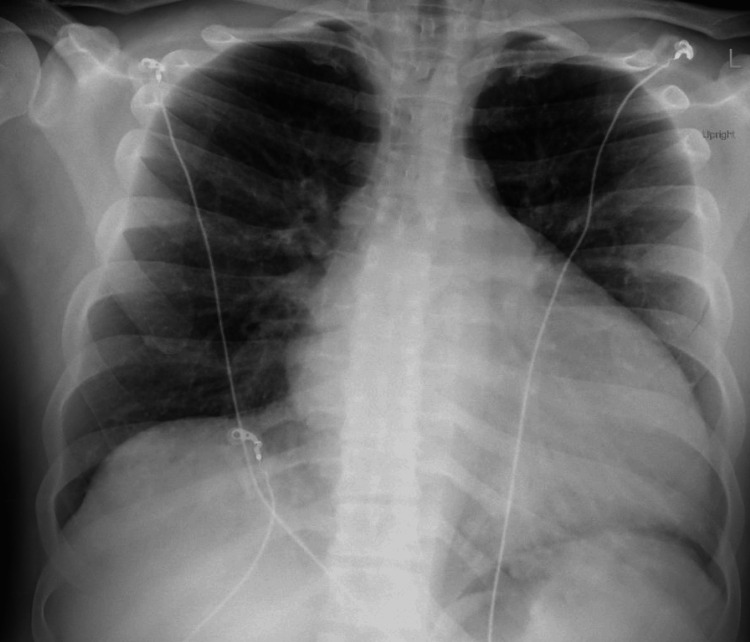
Chest X-ray showing cardiomegaly without effusion.
